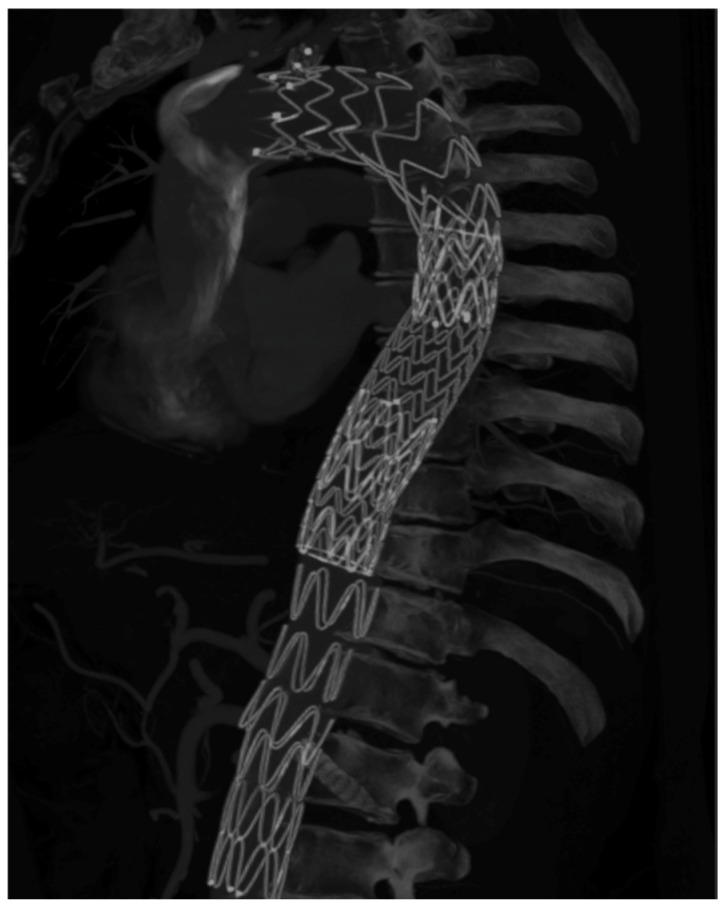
Postoperative 3D CTA reconstruction following Castor implantation combined with the STABILISE technique.
